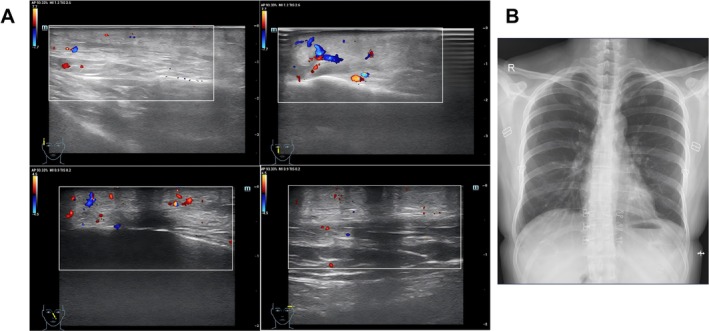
High‐frequency ultrasound images of the swollen facial filler‐injected regions (A) and chest radiography (B).
